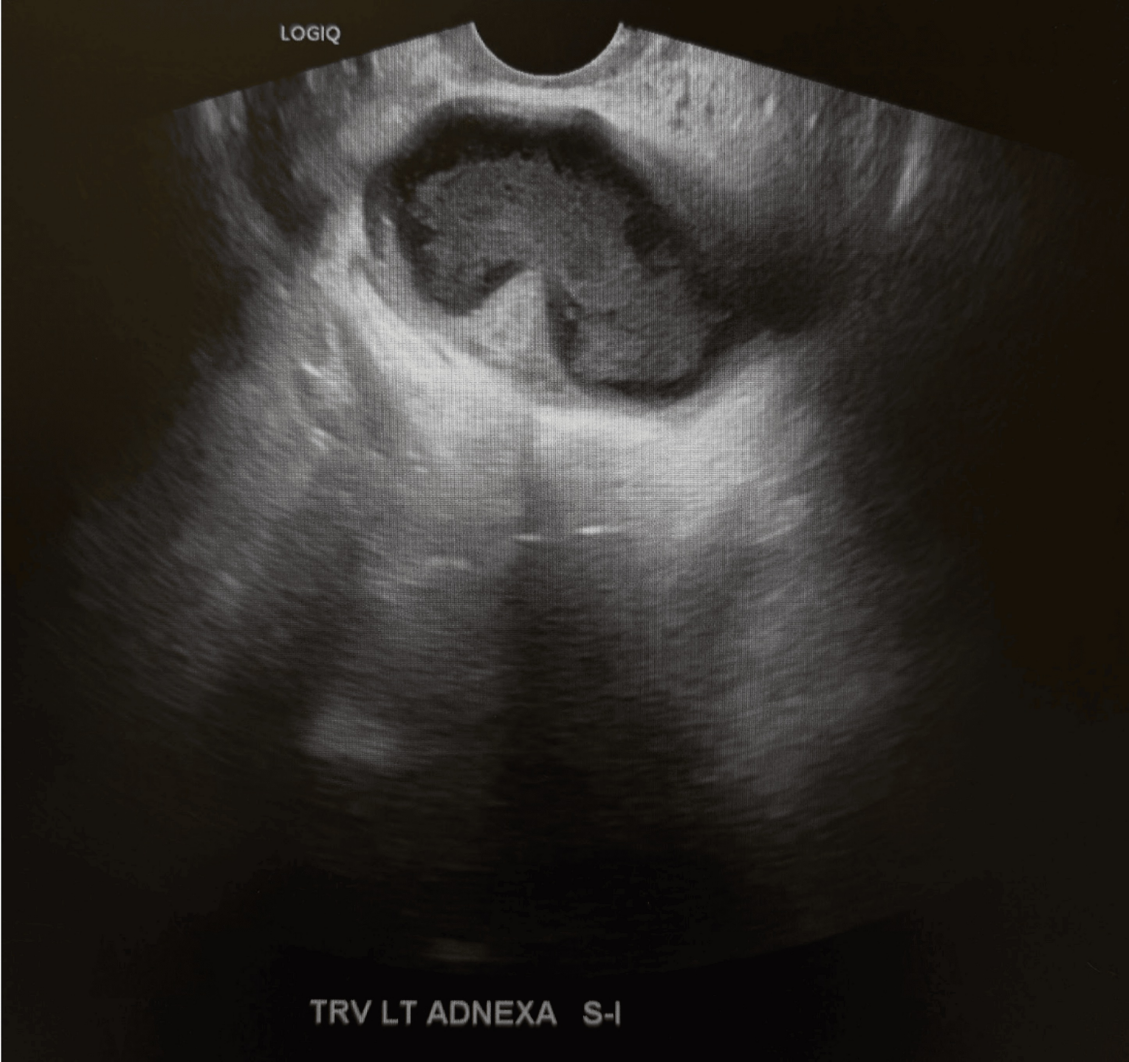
Transvaginal pelvic ultrasound with Doppler image Transvaginal pelvic ultrasound with Doppler showing a complex cystic structure with a tubular configuration containing material measuring 12 x 7.3 x 10 cm, suggestive of a potential hydrosalpinx or pyosalpinx.
